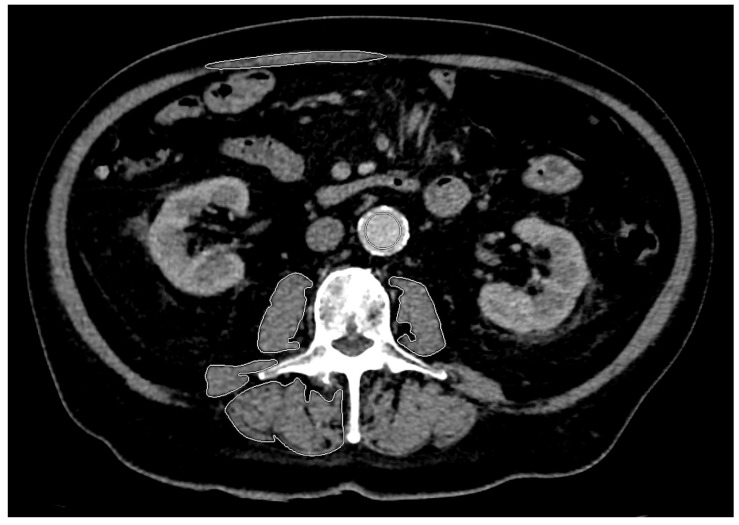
Axial CT at the L3 level and segmentation of the bilateral psoas major muscle, the right erector spinae muscle, quadratus lumborum muscle and rectus abdominis muscle. The latter was excluded from further analysis due to the high variance in segmentation. This semi-automatic segmentation of skeletal muscles was performed using mint Lesion™ software (version 3.8.4, mint Medical GmbH, Heidelberg, Germany). CT: computed tomography; L: lumbar.
